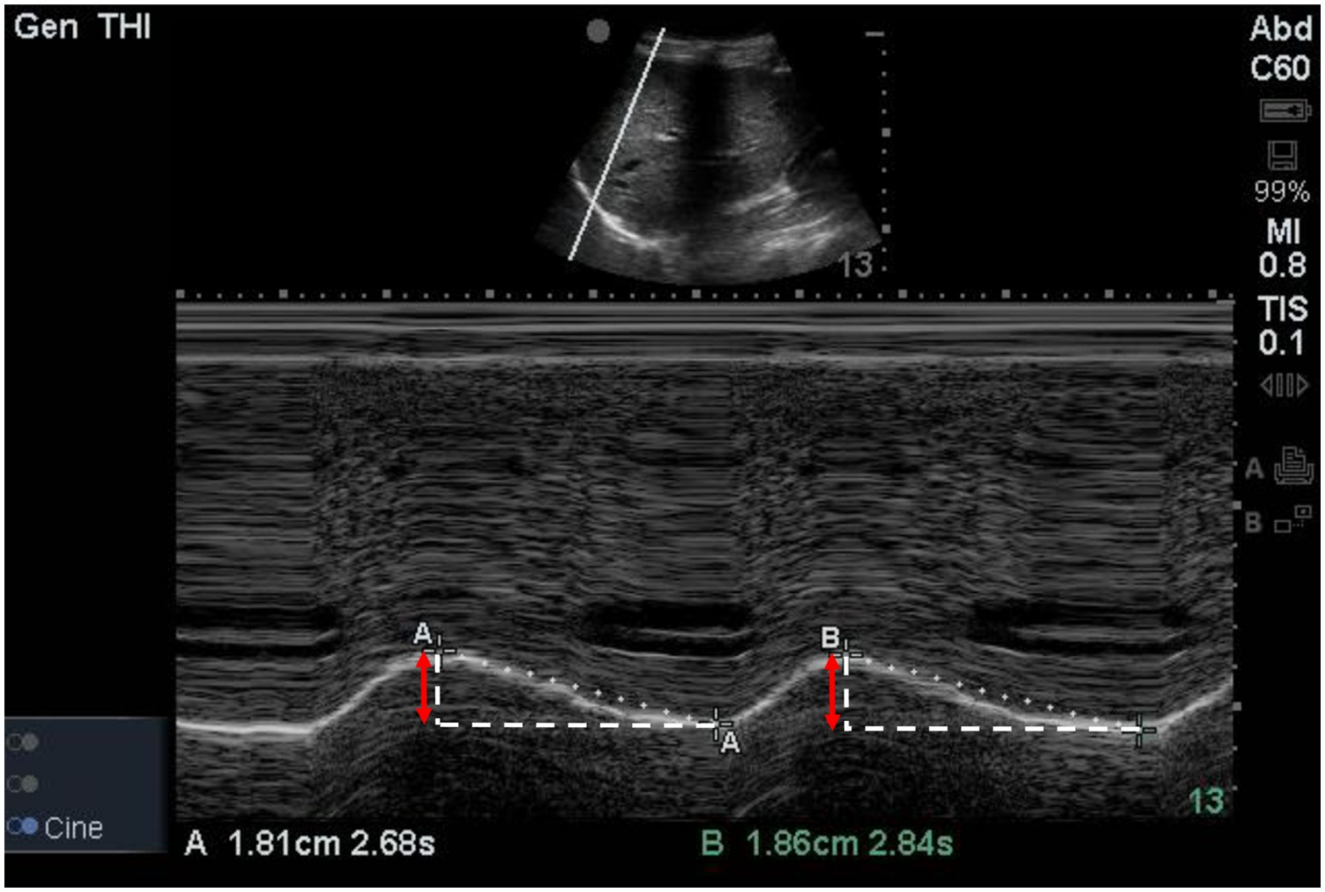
M-mode sonography of diaphragmatic excursion. The amplitude of excursion (red arrow) was measured on the vertical axis of the tracing from the baseline to the point of maximum height of inspiration.
